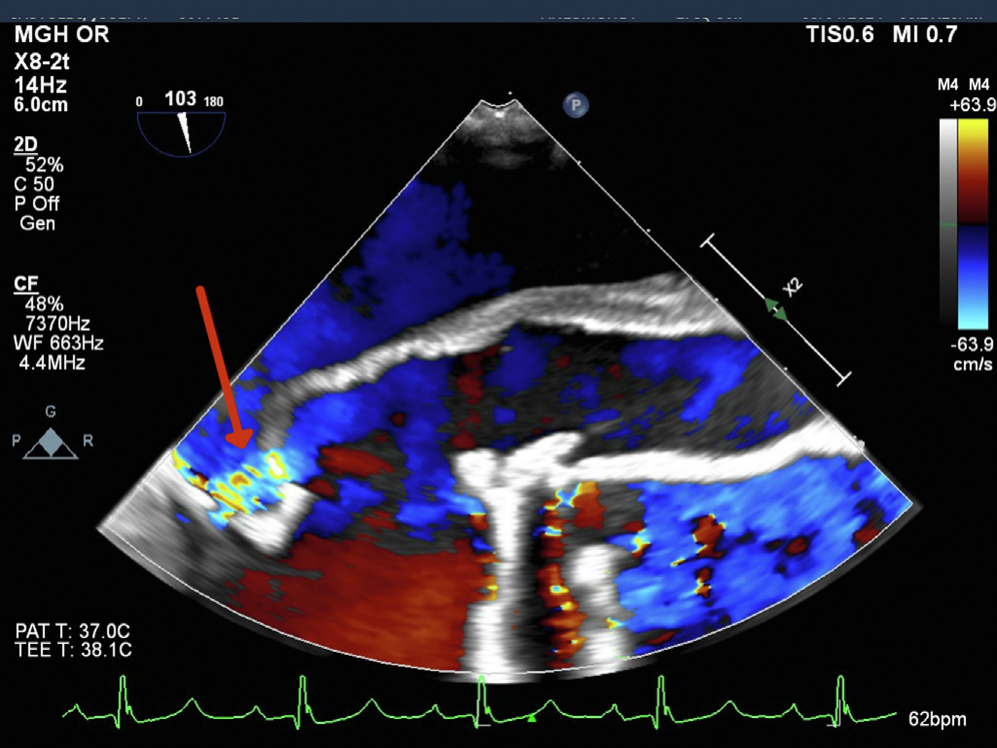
Transesophageal Echocardiogram Displaying Systolic Flow Through Pseudoaneurysm to Left Atrium Transesophageal echocardiogram demonstrating systolic blood flow from the left ventricular outflow tract, through the pseudoaneurysm, and into the left atrium. The red arrow marks the site of the pseudoaneurysm.
